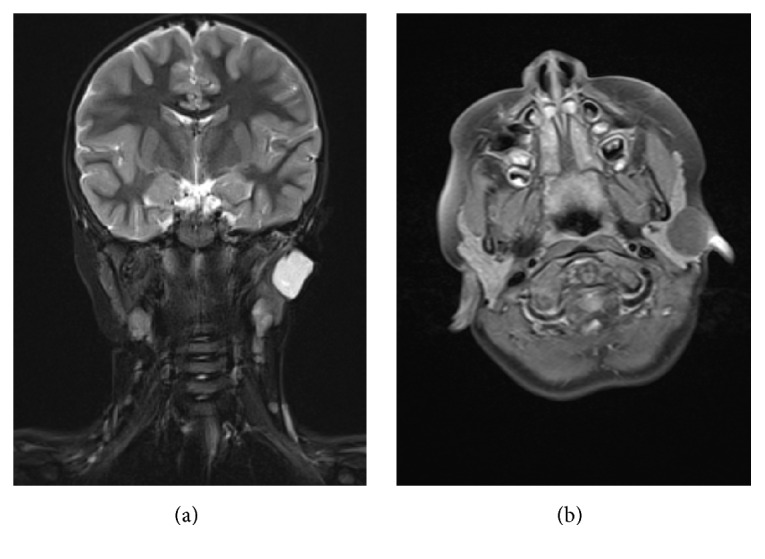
(a) Coronal MRI scan showing a signal enrichment in T2 weighting in the left parotid gland. (b) T1 weighted axial MRI scan showing a hypointensity of the same structure.
